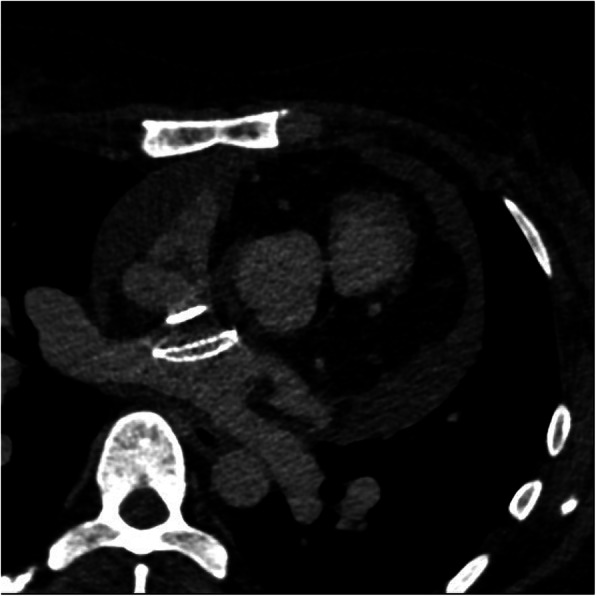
CT angiography showed that ASO device was in proper position through the interatrial septum and there was a retroaortic hematoma due to cardiac erosion
